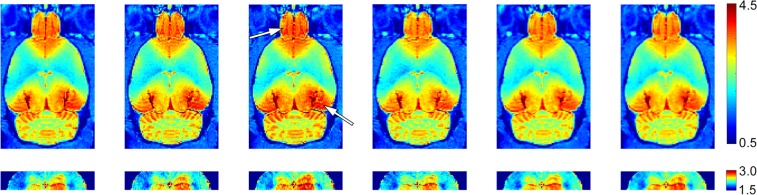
Sequential (left-to-right) T1-weighted images showing increasing Gadovist concentration in the olfactory bulb and hippocampus. The hippocampus is shown with increased contrast below. White arrows indicate regions of maximum signal intensity.
